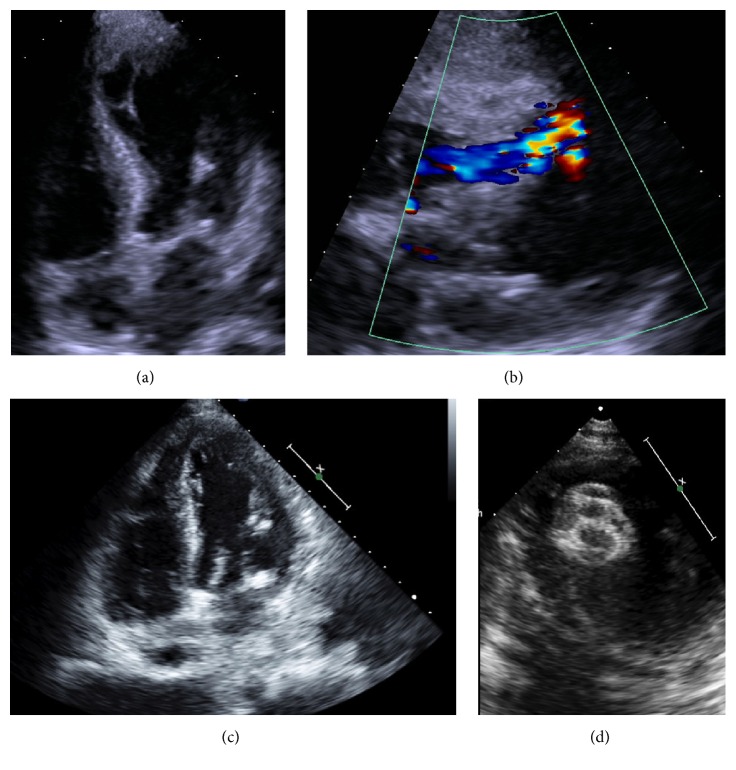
(a) Outside hospital TTE. Apical 4 chamber view demonstrating left ventricular apical ballooning. (b) Outside hospital TTE. Subcostal view demonstrating VSD with left to right shunt. (c) TTE 11 days later with recovery of left ventricular morphology. (d) Amplatzer in place after repair of VSD.
